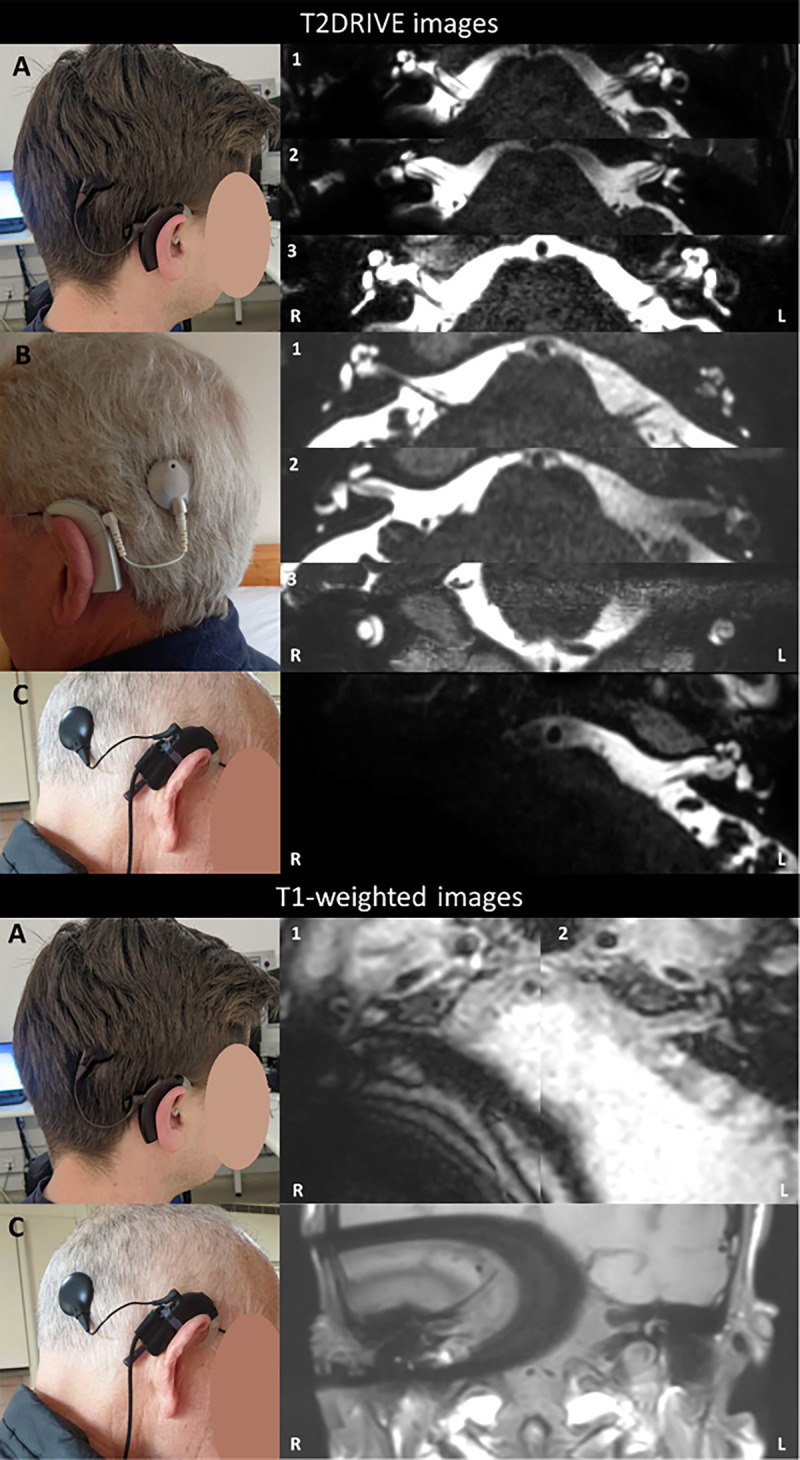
Top*, T2DRIVE images in three CI users (A, B, and C). In participant A, transverse slices through the bilateral IACs are shown without MARS (1 and 2) and with MARS (3). In participant B, transverse (1 and 2) and coronal (3) are shown. In participant C, a transverse slice is shown. *Bottom*, T1-weighted images are shown in two CI users (A and C). In participant A, transverse slices through the bilateral IAC are acquired using a T1-weighted TSE with mDIXON and no metal artifact reduction. In participant C, a transverse slice is shown, acquired using a T1-weighted TSE with SPIR and SEMAC.
